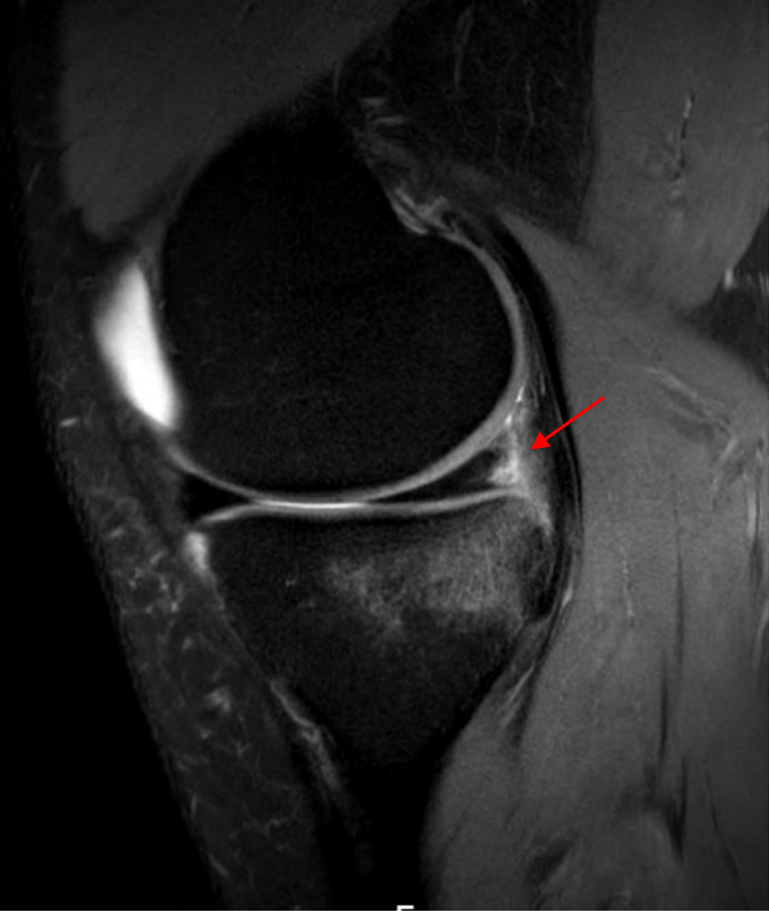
Meniscal ramp lesion type 4B (complete meniscocapsular junction tear) (arrow) has shown by the fluid intensity signal extending from the superior to the inferior articular surface on sagittal proton density fat saturation magnetic resonance imaging sequence (right knee)
